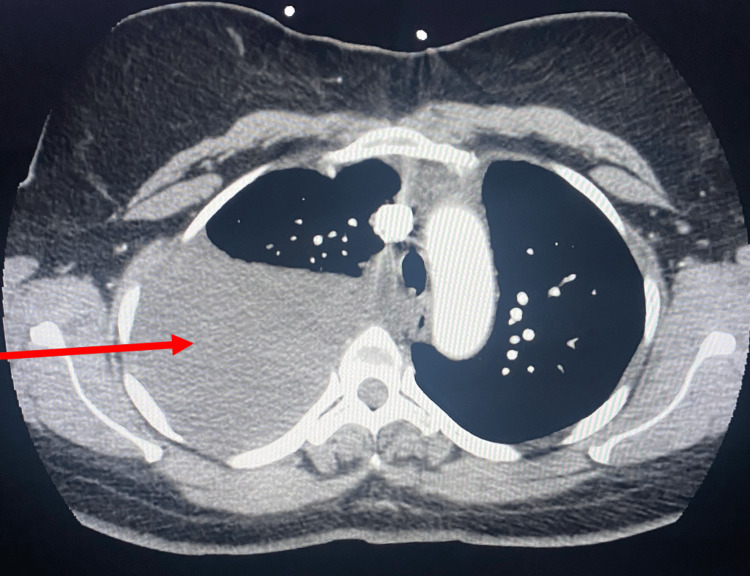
CT Angiography Chest Showing Re-accumulation of Large Right-Sided Pleural Effusion Red arrow highlighting the area of interest. CT: computed tomography
